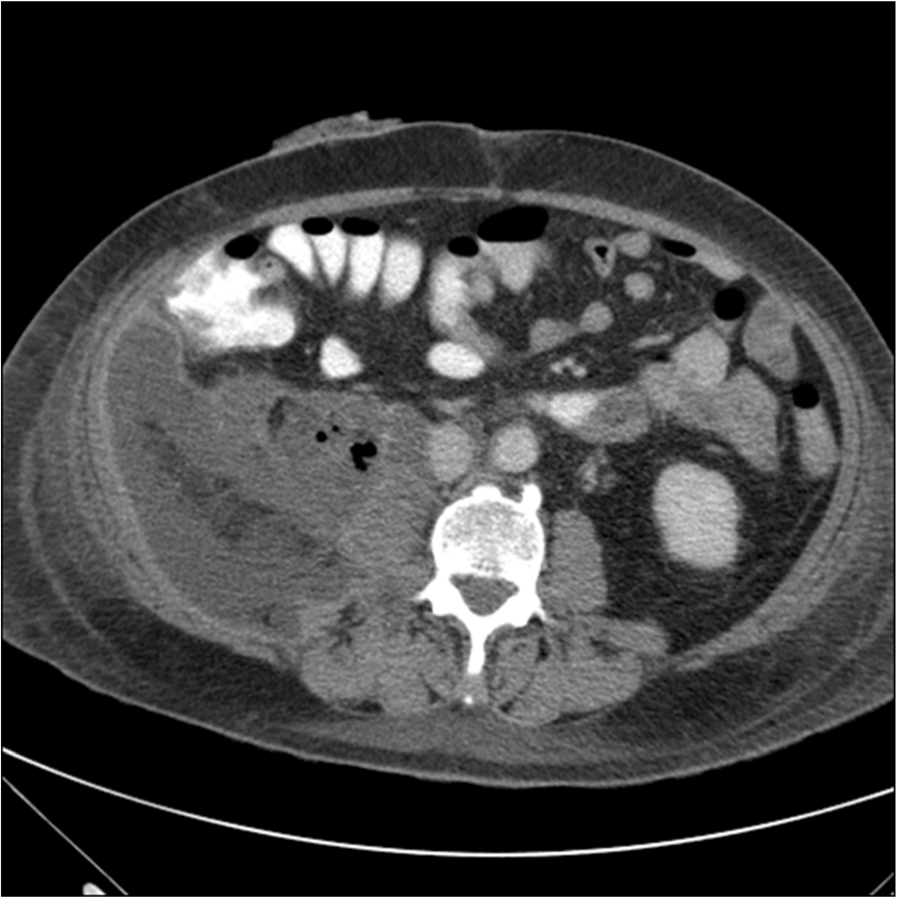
CT image showing extensive retroperitoneal necrosis prior to surgical intervention (Case 2).
